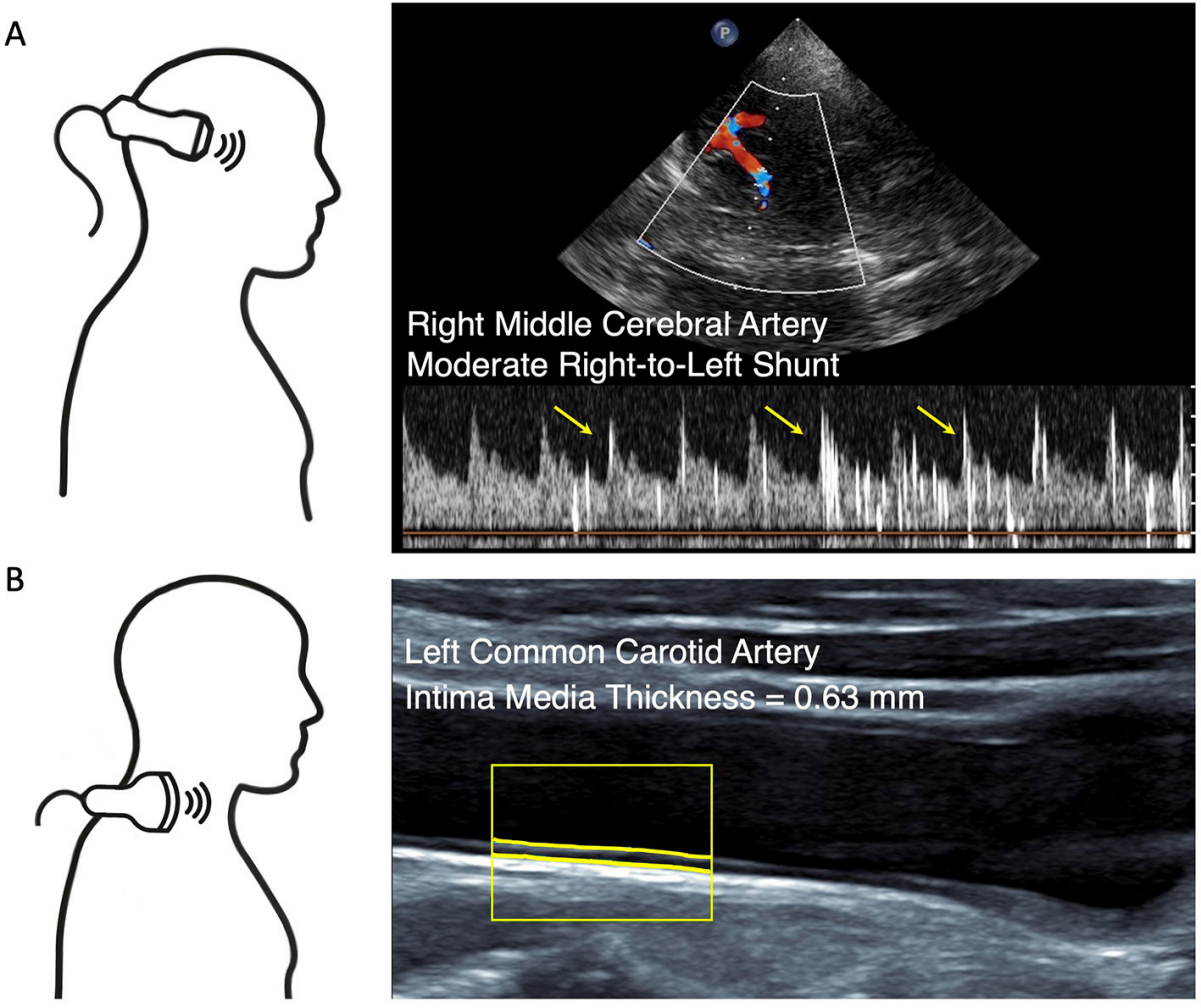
Non-invasive ultrasound. (A) Transcranial Doppler (TCD) bubble test at rest. Duplex-guided Doppler velocity spectrum of the right middle cerebral artery reveals moderate right-to-left shunt within 14 s after the injection of agitated saline. Yellow arrows point to exemplary high-intensity signals. (B) Carotid ultrasound with software delineation of the intima media complex reveals normal intima media thickness (IMT).
